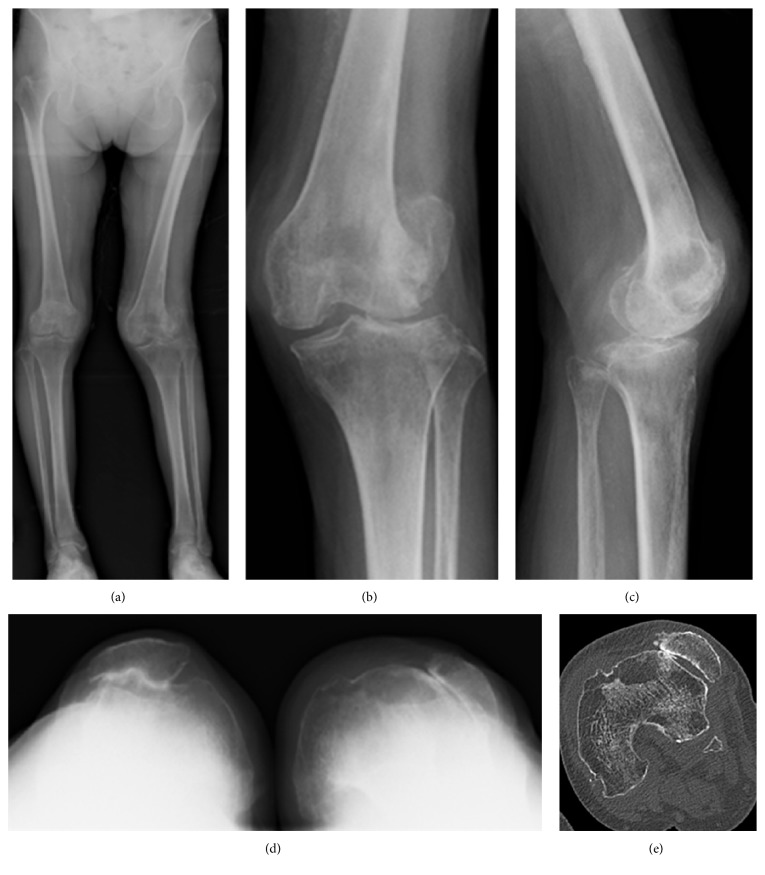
Preoperative plain radiograph and computed tomography. Anteroposterior long leg standing image of both legs (a), anteroposterior one leg standing image of the left knee (b), lateral one leg standing image of left knee (c), skyline view of patella at 30 degrees of knee flexion (d), and preoperative computed tomography of the left knee (e).
